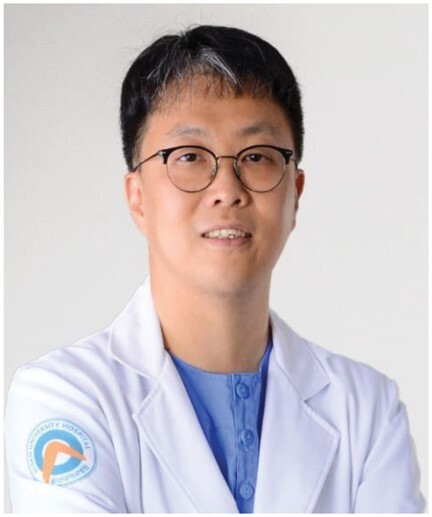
Ki-Bum Won is Assistant Professor of Cardiology at Ulsan University Hospital, University of Ulsan College of Medicine, South Korea. Dr. Won is an expert in cardiac computed tomography and coronary artery disease. He has been studying metabolic syndrome, diabetes mellitus, and atherosclerosis with non-invasive imaging tools and biomarkers focusing on the primary prevention for over ten years. Dr. Won has published about 40 research papers as first or corresponding author in the field of atherosclerotic cardiovascular disease.
